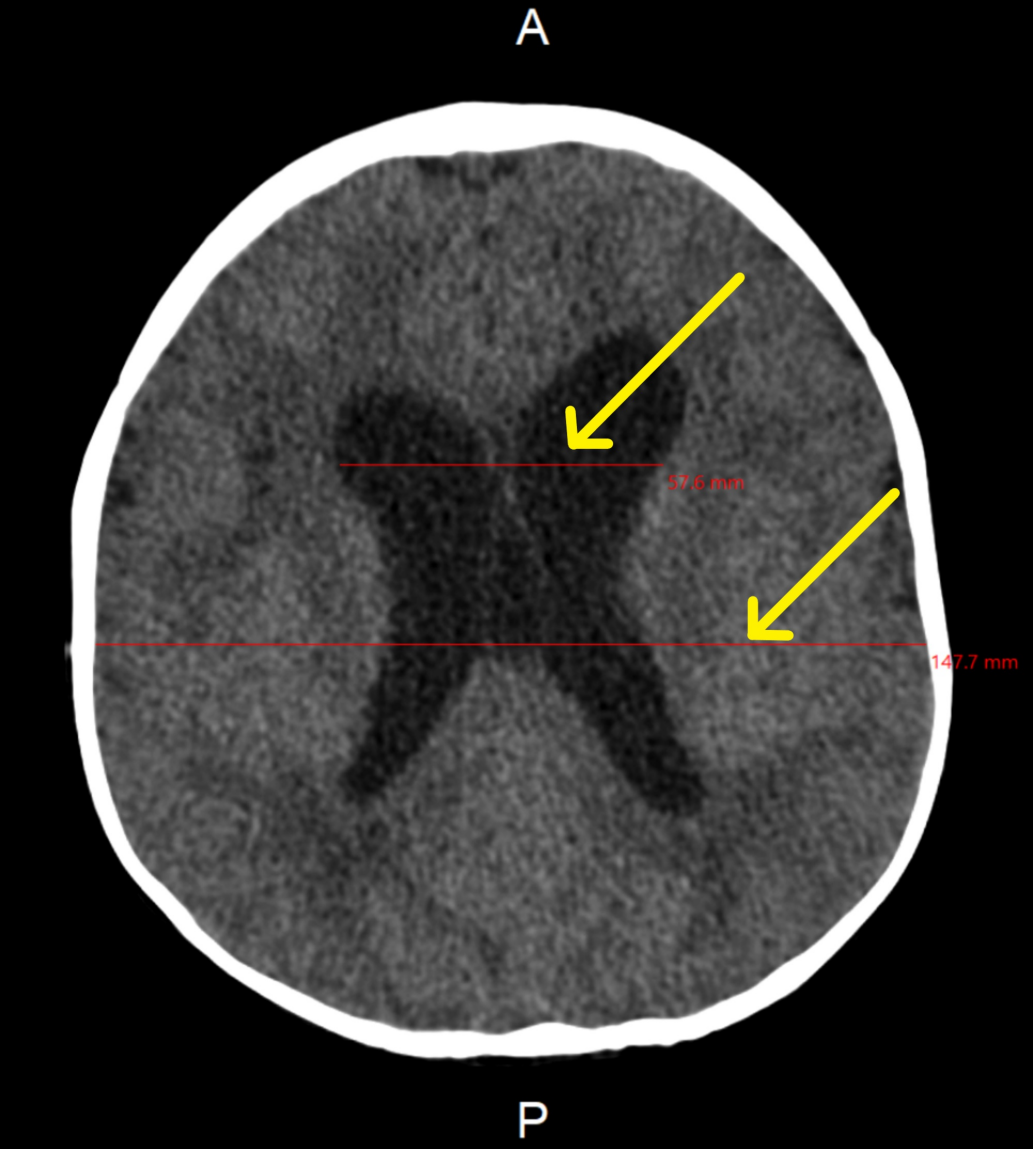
Brain CT showing ventricular dilation
